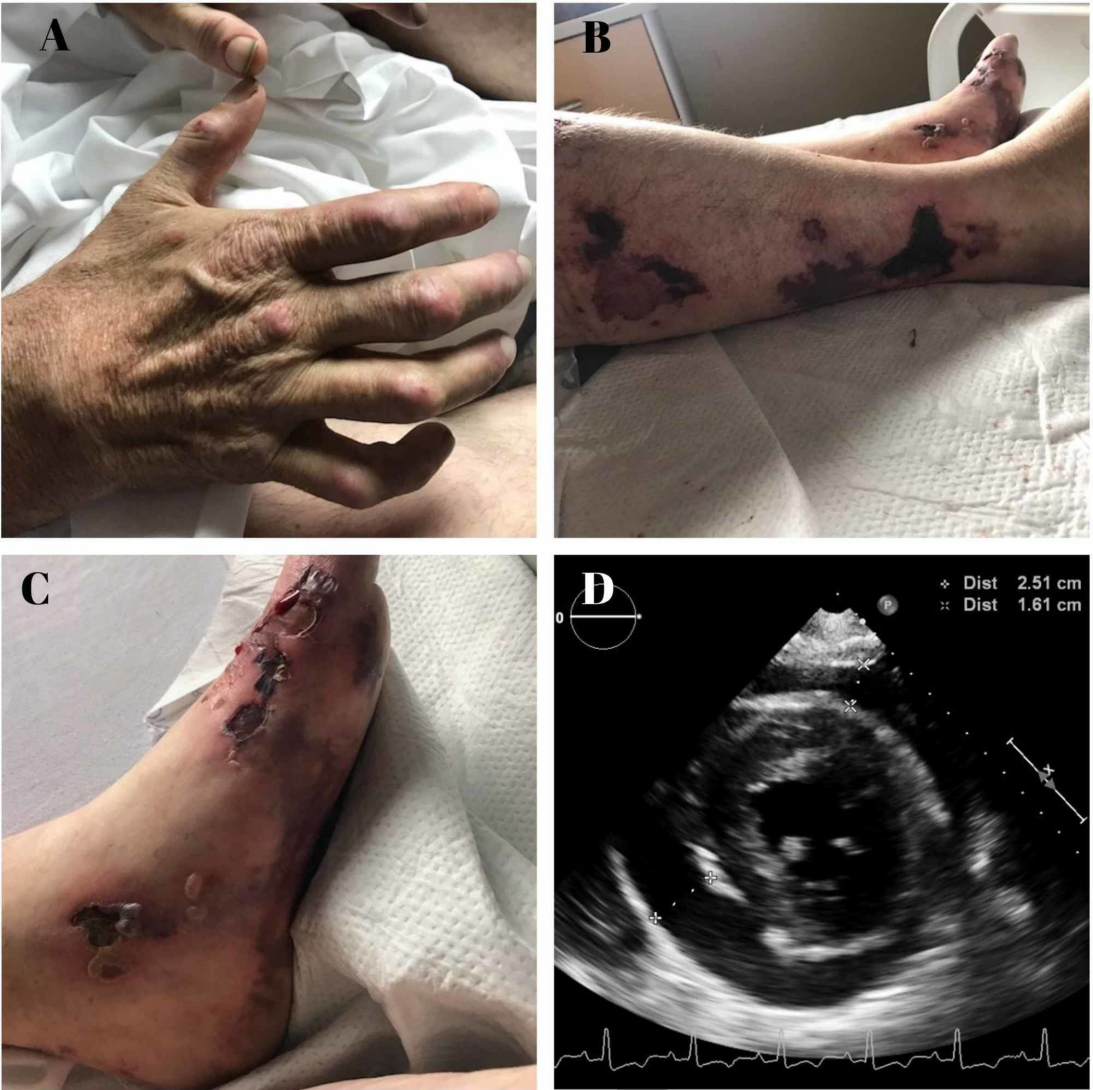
(A) Rheumatoid arthritis involving hands. (B & C) Skin manifestations of rheumatoid vasculitis. (D) Transthoracic echocardiogram showing large pericardial effusion.
